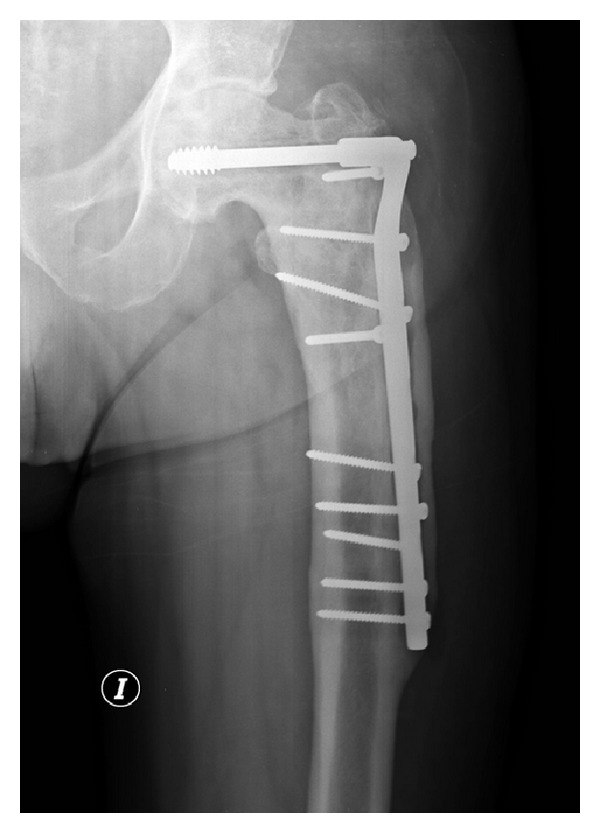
Preoperative AP radiograph of the left hip showing a healed intersubtrochanteric fracture with a dynamic condylar screw and osteosynthesis plate, coxa vara, and posttraumatic osteoarthritis.
